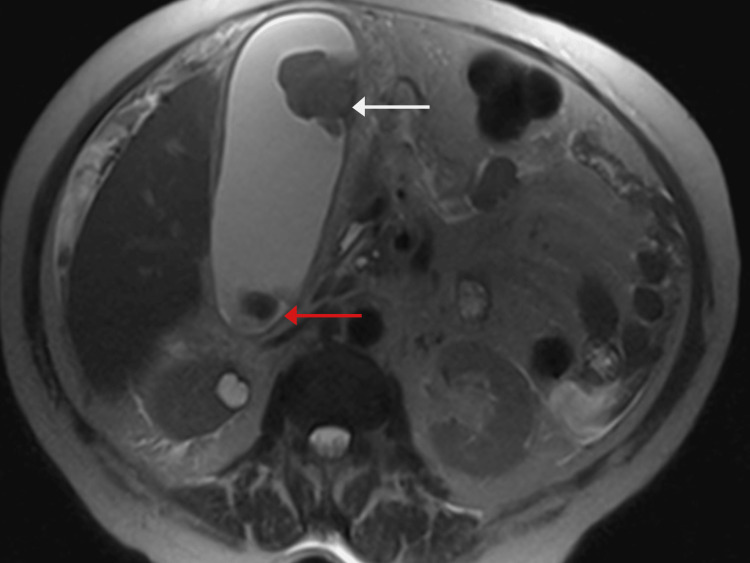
MRI demonstrating a large mass within the gallbladder (white arrow) and one of the two gallstones (red arrow) identified.
